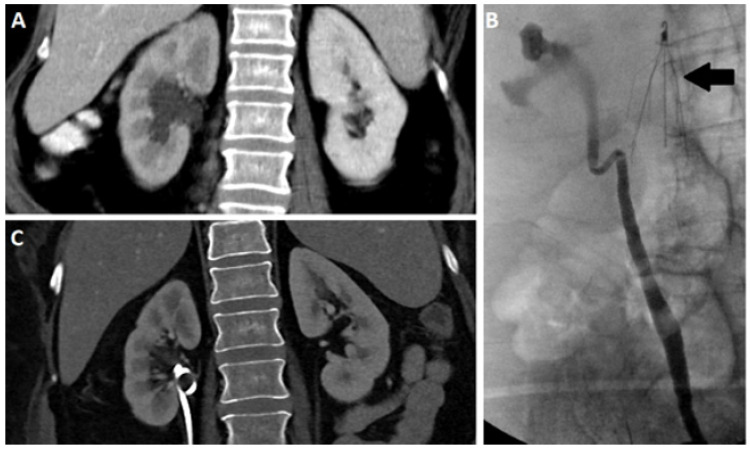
Coronal contrast enhanced CT (A) and retrograde pyelogram (B) depicting a hydronephrotic right kidney. Coronal CT after nephroureteral stent (C) placement demonstrating resolution of hydronephrosis. Note the presence of an IVC filter (arrow).
